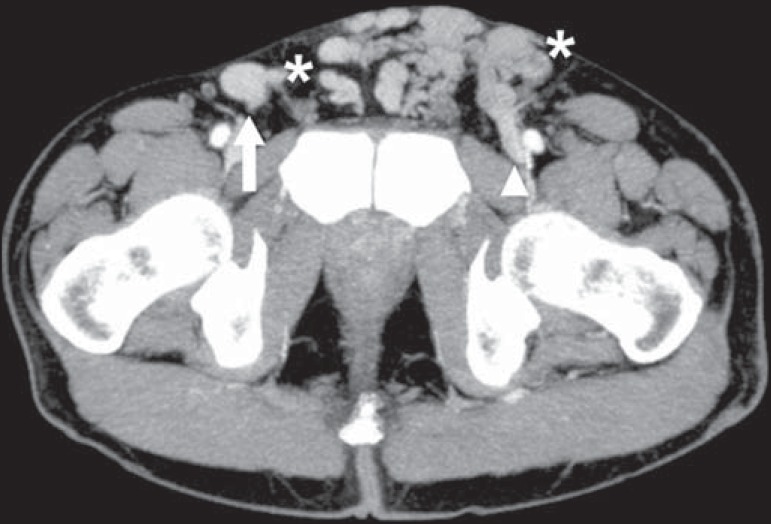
Cockett syndrome or May-Thurner syndrome. Axial CT showing suprapubic
